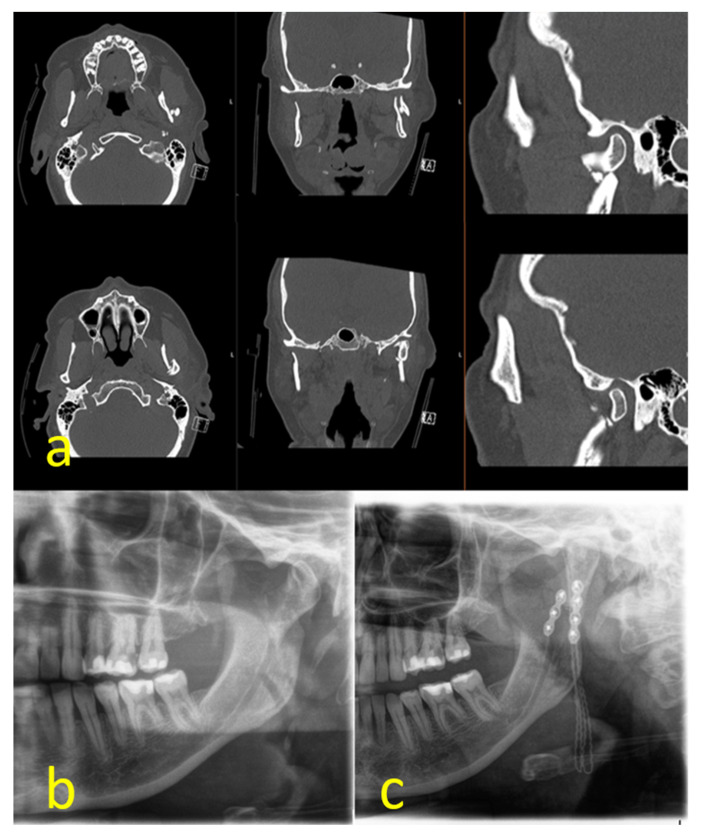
Comminuted type II fracture: CT-scan (a); preoperative panoramic x-ray (b); postoperative panoramic x-ray (c).
